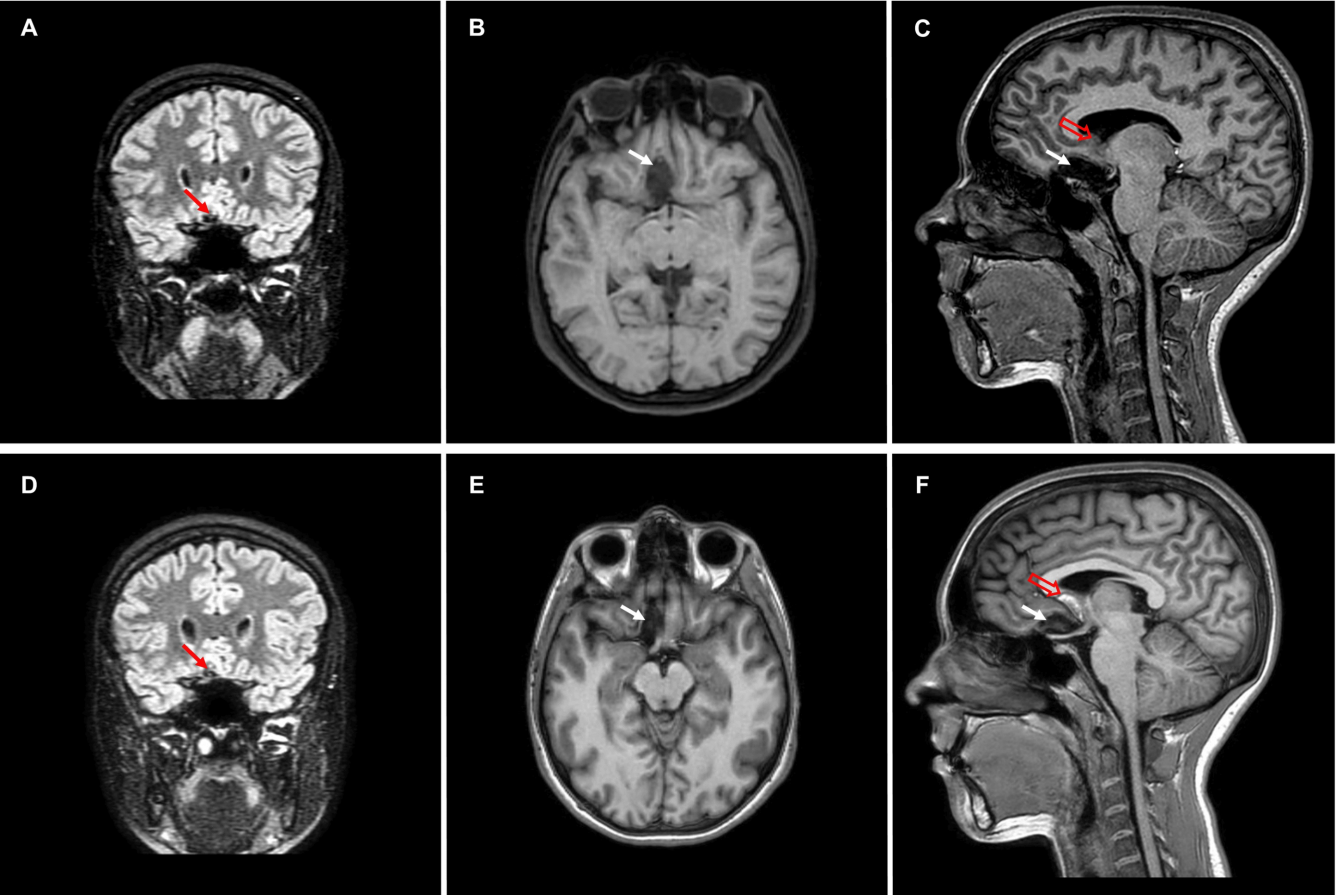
Postoperative MRI progressive changes within the surgical cavity (full arrows) and a slight decrease of the subrostral component under the corpus callosum (empty arrows) (A) One-year postoperative FLAIR coronal view, (B) one-year postoperative T1 axial view, (C) one-year postoperative T1 sagittal view, (D) two-year postoperative FLAIR coronal view, (E) two-year postoperative T1 axial view, and (F) two-year postoperative T1 sagittal view FLAIR: fluid-attenuated inversion recovery
